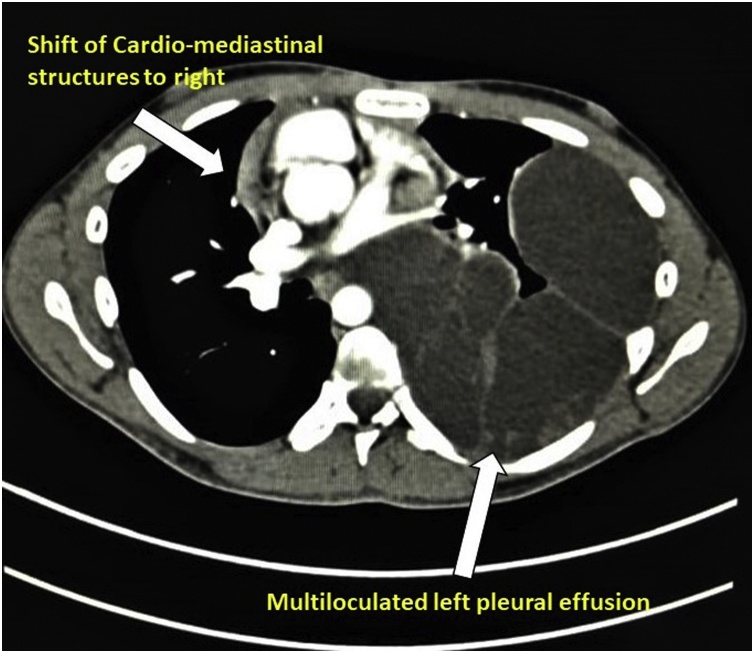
PET CT done post chemotherapy showed “considerable increase in cardiomediastinal structures with persistent multiloculated pleural effusion”.
